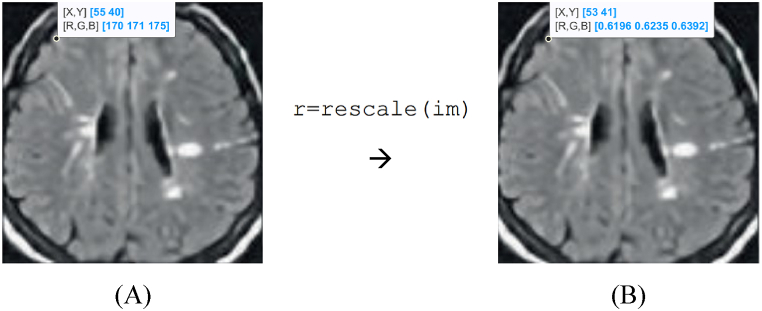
Effect of min-max normalization on brain MRI images: (A) original image, and (B) image after min-max normalization.
